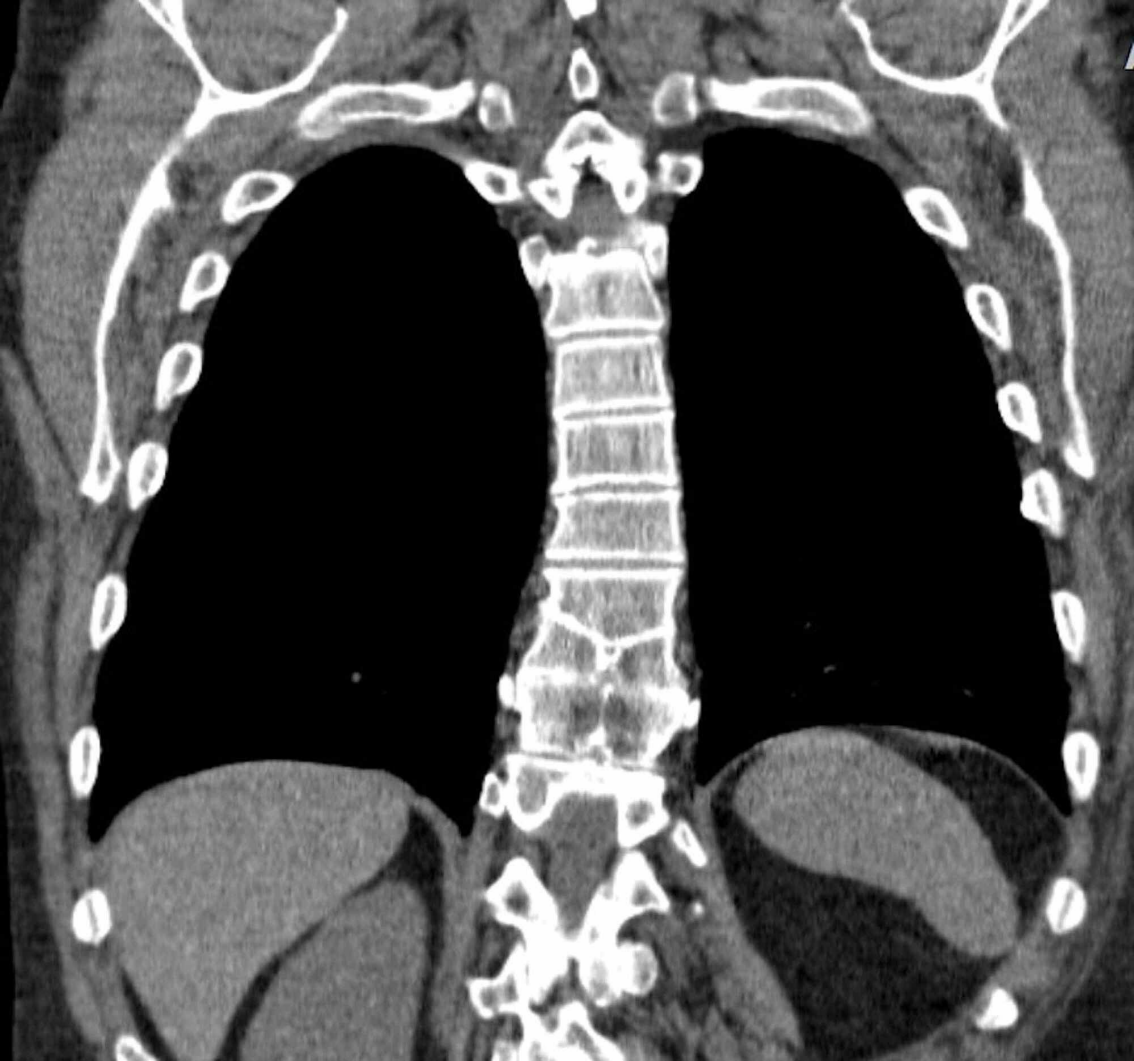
CT of the chest without contrast, coronal view. This view demonstrates a T10 butterfly vertebra as well as fusion of T9-T10 and T10-T11.
